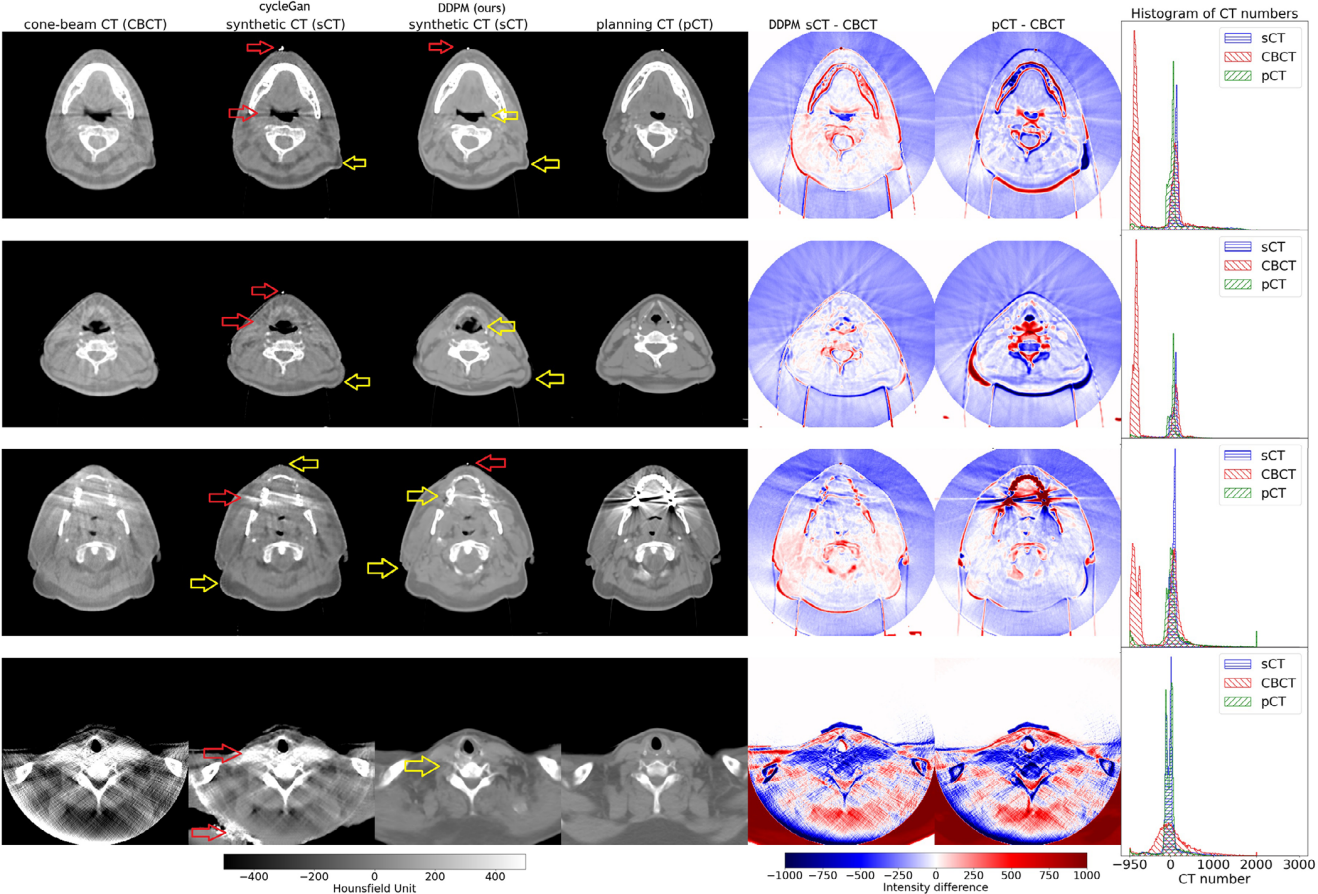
Samples of our DDPM‐generated sCT with the guiding CBCT and corresponding pCT images, HU difference maps, and histogram of CT numbers for CUH patient test images. Samples of cycleGAN‐generated sCTs were also displayed for comparison. Improved sCT features were annotated with yellow arrows, while poor‐performing sCT features were annotated with red arrows. Note: histogram was truncated to −950 HU at the lower limit to exclude air, which would otherwise overwhelm other HU values.
